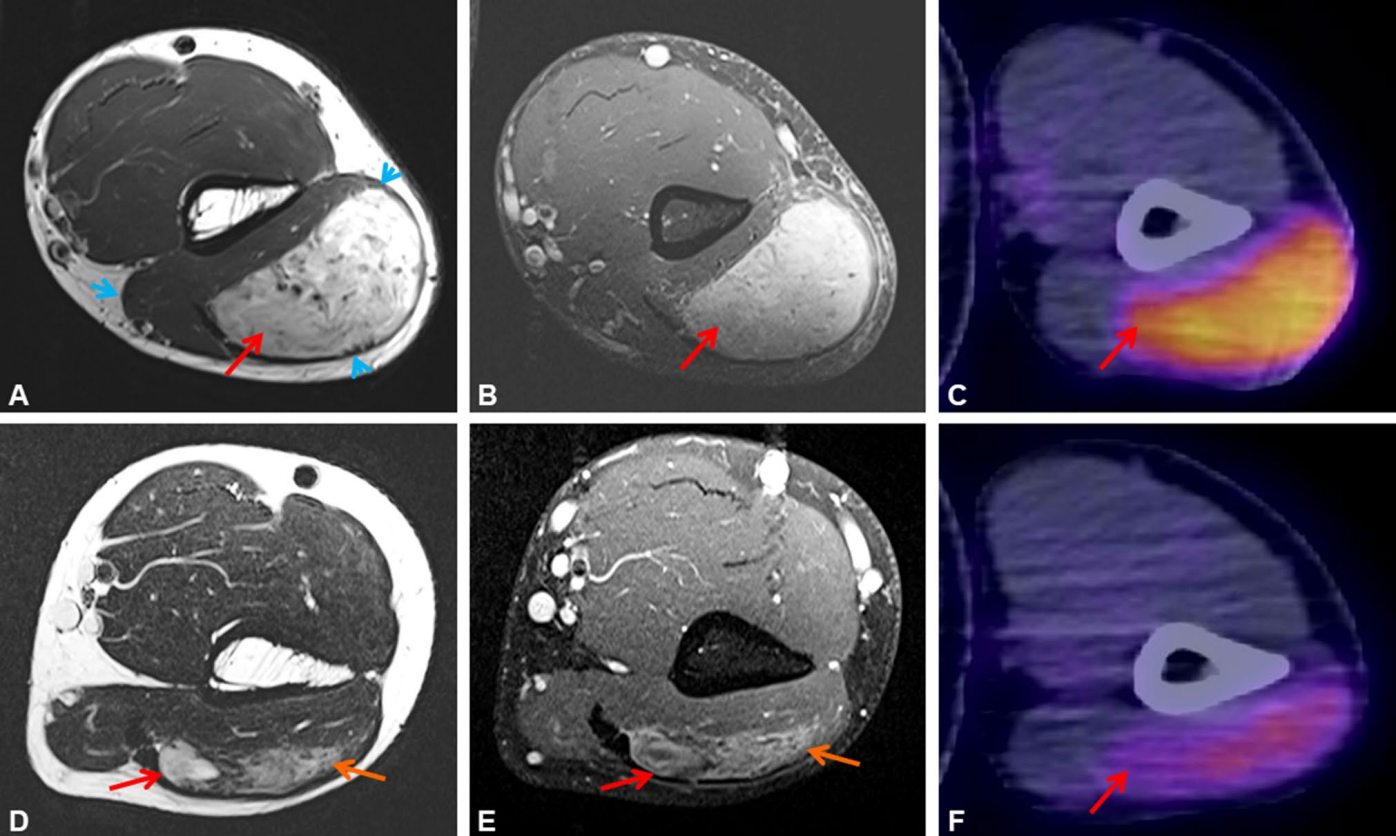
Well-defined mass (red arrow) within the left triceps brachii muscle (blue arrowheads). The mass is hyperintense on T2-weighted MR imaging (A) and shows strong contrast enhancement (T1-weighted image) (B). MR imaging performed 28 days after treatment initiation. Further shrinkage of the tumor, with solid (red arrow) and diffuse (orange arrow) residual areas (D). Remaining mild, inhomogeneous contrast enhancement (red  and orange arrow) (E). ^18^F-FDG-PET/CT on initial presentation reveals glucose hypermetabolism of the lesion (SUV_max_ 6.6) (C). ^18^F-FDG-PET/CT performed 12 days after treatment initiation with selpercatinib. Markedly reduced glucose hypermetabolism of the tumor (red arrow; SUV_max_ 2.9, SUV_max_ of the triceps brachii muscle 0.9) (F)
